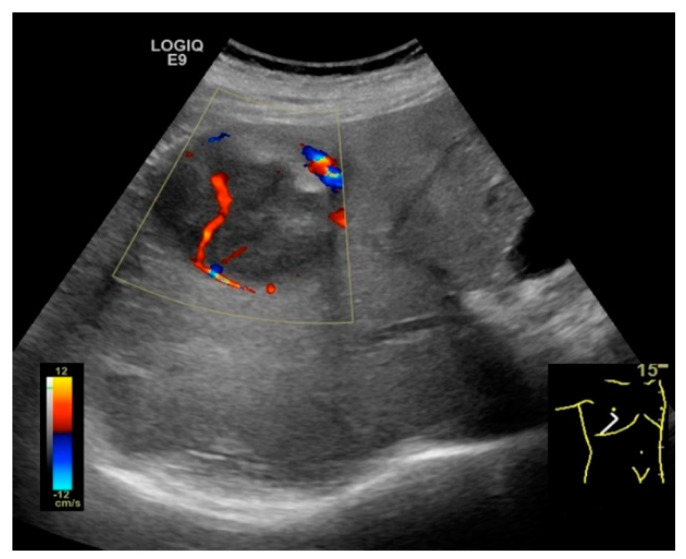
Hepatic steatosis. Focal liver lesion. 2D-US scan shows an enlarged liver with increased echogenicity and posterior beam attenuation. In addition, a focal parenchymal structure is observed in the right lobe. It is characterized as having decreased, heterogeneous echogenicity and internal vessels seen at Doppler examination. The diagnosis of the focal lesion remains uncertain and CEUS examination is necessary.
